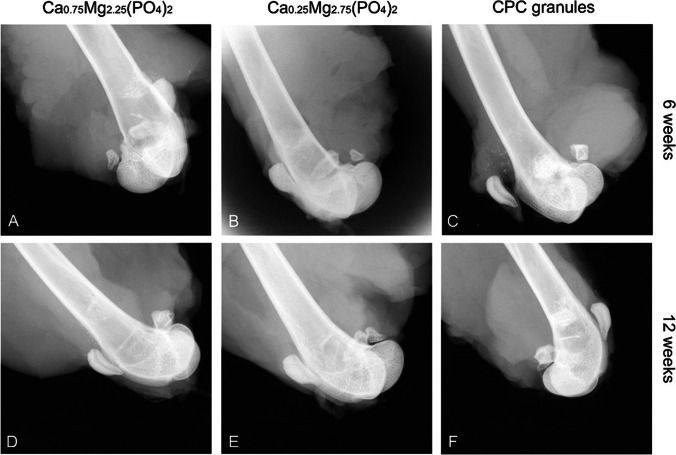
Radiographs of the implanted granules in the distal femoral epicondyle after explantation
